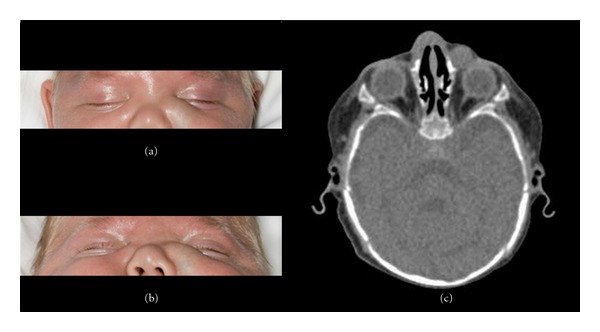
(a) and (b) Nonerythematous, bluish lesion 1.5 × 1.0 cm below the nasal lower lid. (c) CT scan showing 11 × 9 mm lesion along left nasocanthal fold.
